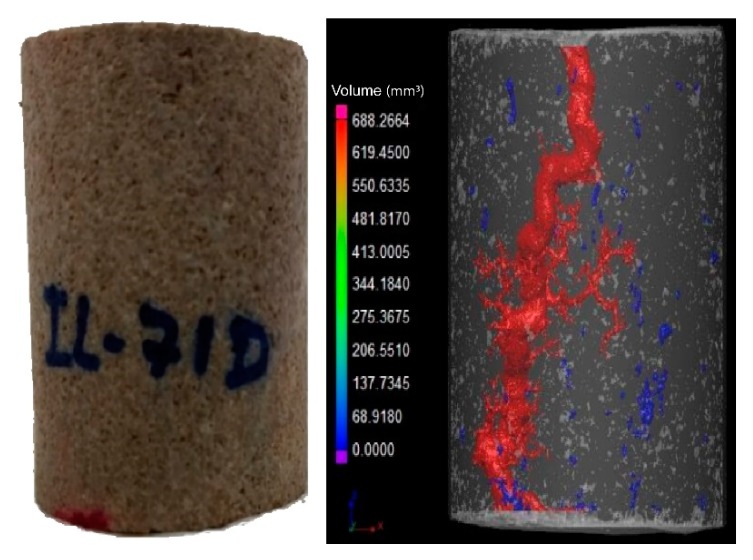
Imaging of wormhole structure using CT scan; ANS1; 0.5 mL/min (IL-71D).
